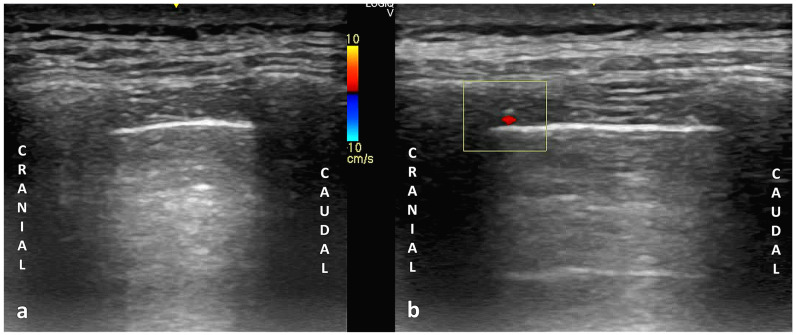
Sonographic visualization of the intercostal artery with a high-frequency linear probe: (a) B-mode image of the intercostal space acquired in longitudinal orientation (cranial to the left, caudal to the right). (b) Color Doppler image of the same region, showing an arterial Doppler signal (highlighted in the yellow box) within the neurovascular bundle located in the costal groove along the inferior border of the superior rib, consistent with the anatomical course of the intercostal artery.
